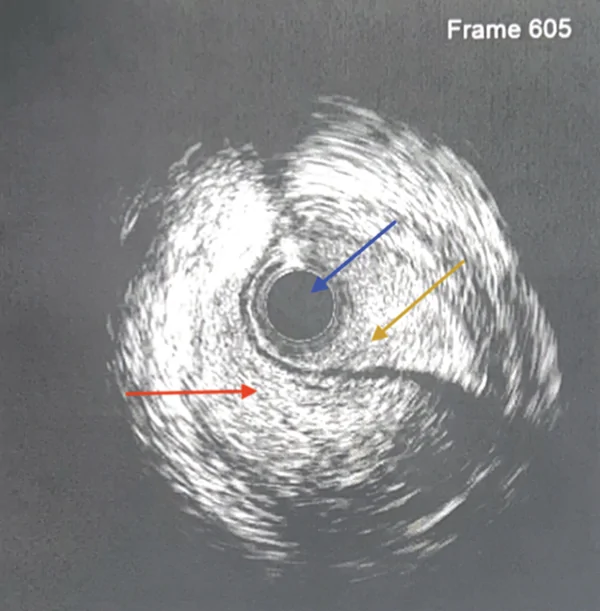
Prestenting intravascular ultrasound (IVUS) image of the left anterior descending artery. IVUS confirmed positioning of the imaging catheter within the distal true lumen and demonstrated an eccentric intramural hematoma with associated true‐lumen compression before stent deployment. The blue arrow indicates the IVUS catheter, the yellow arrow indicates the true lumen, and the red arrow indicates the intramural hematoma.
